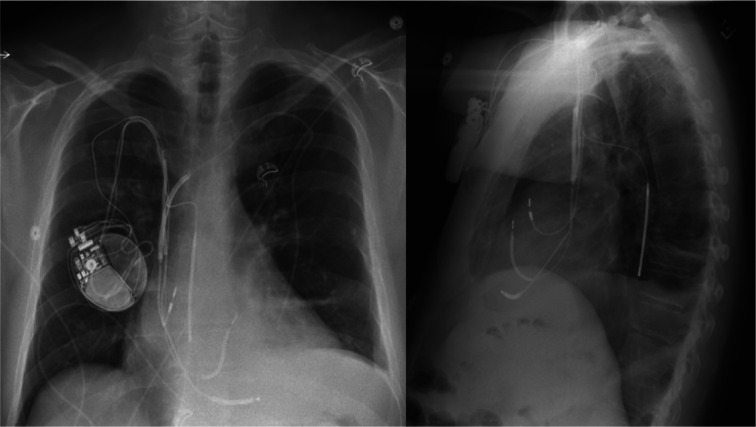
Chest X-rays showing an azygos defibrillator lead located posterior to the left ventricle.
